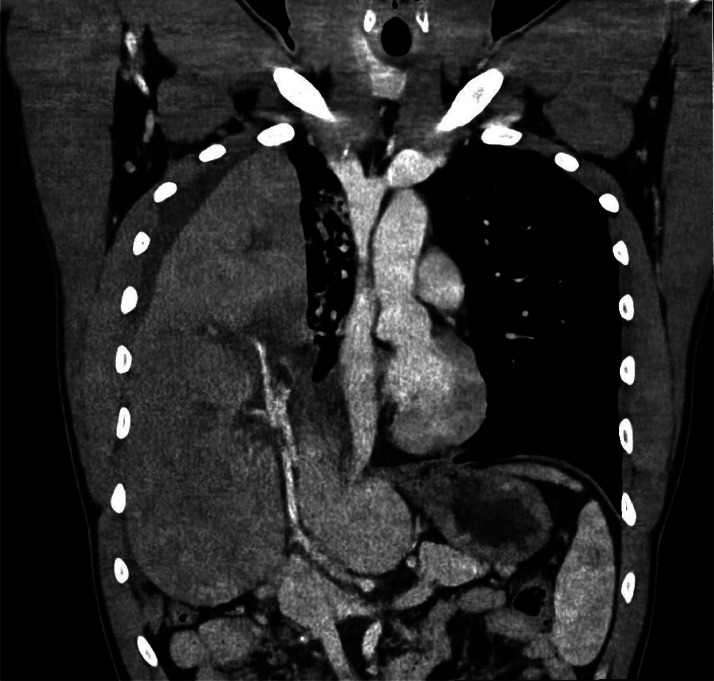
Coronal image from a portal venous CT, showing a complete ascension of the liver into the thoracic cavity, with the left lobe being completely verticalized; signs of hepatic hypoperfusion can also be identified.
